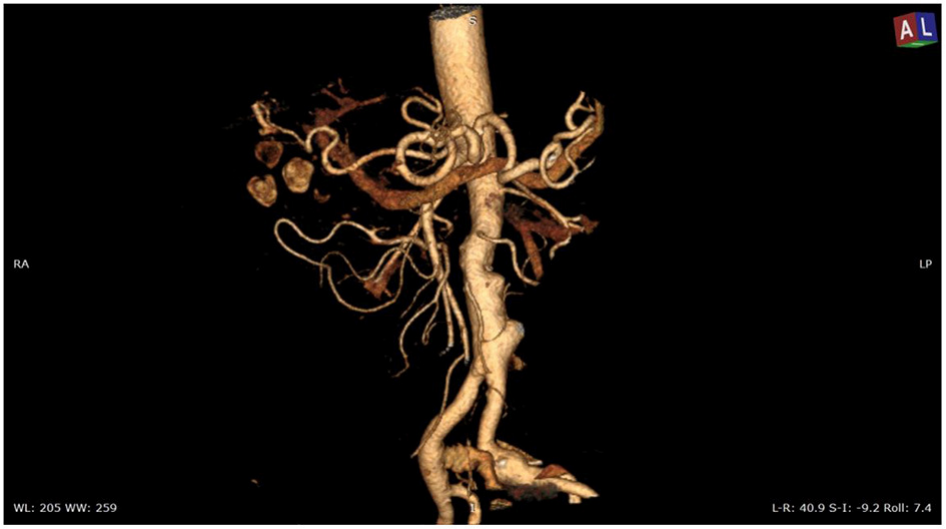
Angio-CT scan of the thoracoabdominal aorta showing aneurysmal degeneration of penetrating aortic ulcers in the infrarenal aorta associated with lithiasis of the gallbladder.
